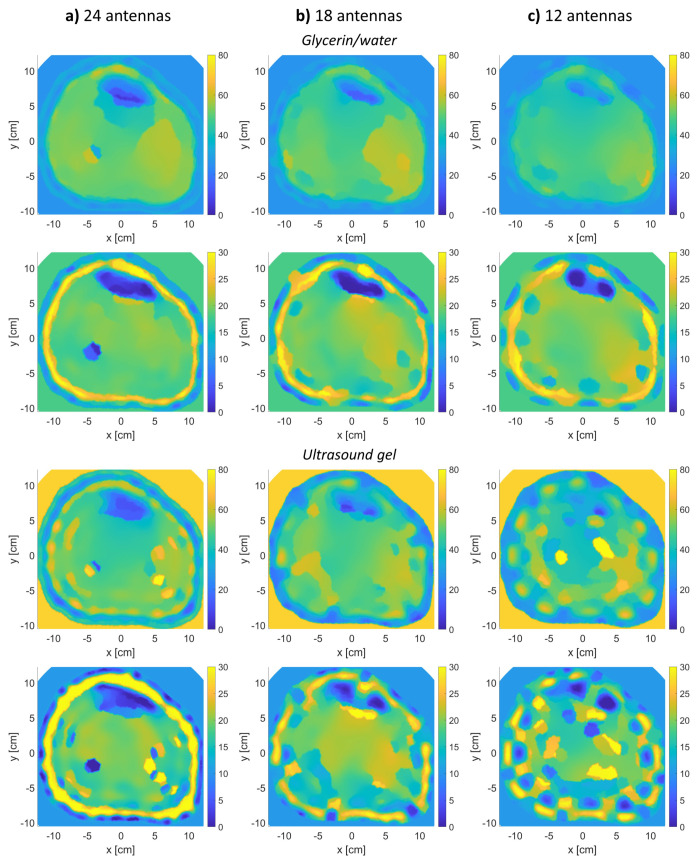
Varying the number of antennas in the reconstruction of leg model 1 using glycerin/water and ultrasound gel matching media: (a) twenty-four antennas, (b) eighteen antennas, and (c) twelve antennas (top row: real relative permittivity, bottom row: imaginary relative permittivity).
